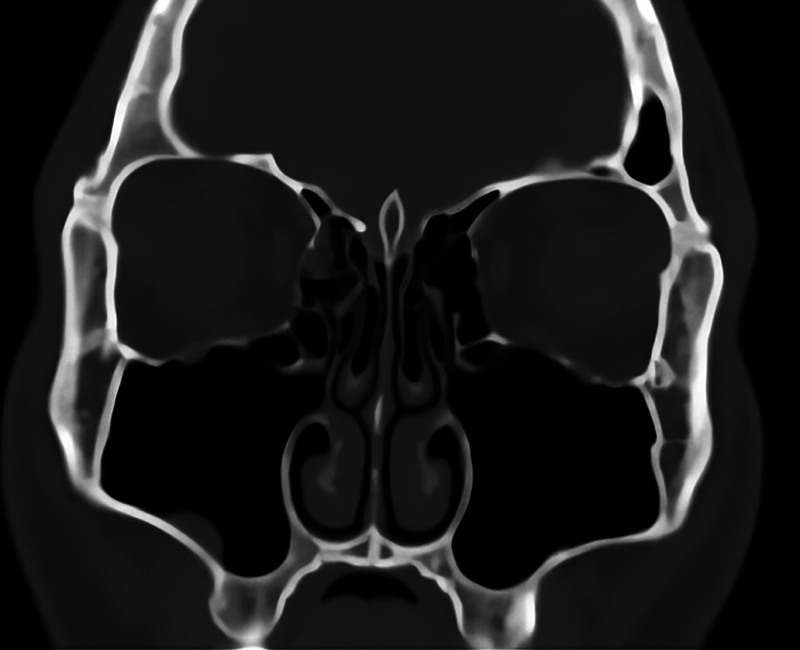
Computed tomography scan showing a pneumatized UP.
